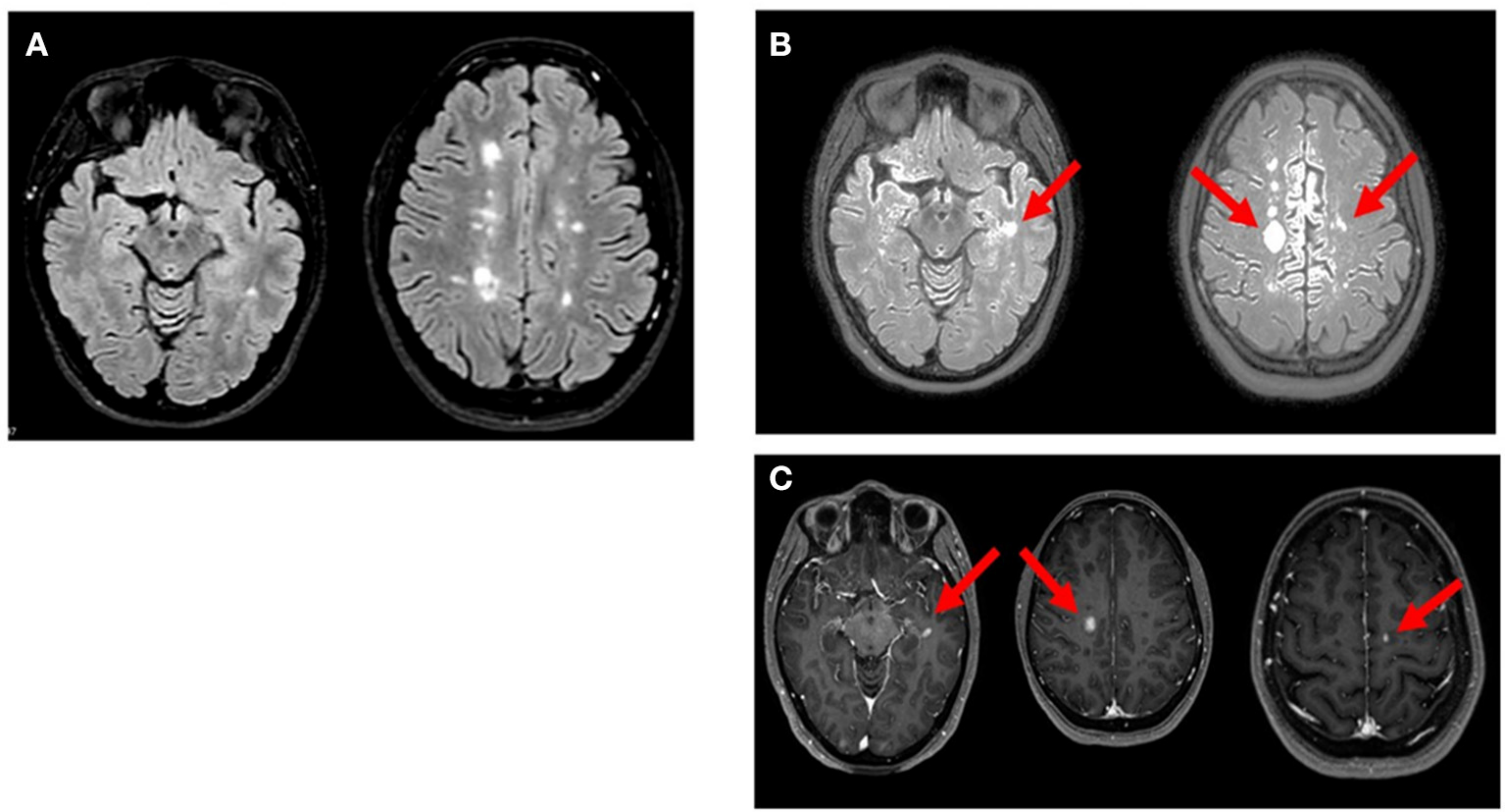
Axial MRI images of reported patient. (A; Oct 2020): multiple hyperintense white matter lesions were observed in T2/FLAIR weighted scan. (B; Apr 2021): T2/FLAIR weighted scan showed new three lesions in left temporal lobe and bilateral precentral cortex (arrows). (C; Apr 2021): gadolinium-enhancing lesions (arrow) corresponding to the new hyperintense lesions on FLAIR were noted in T1 weighted postcontrast images.
